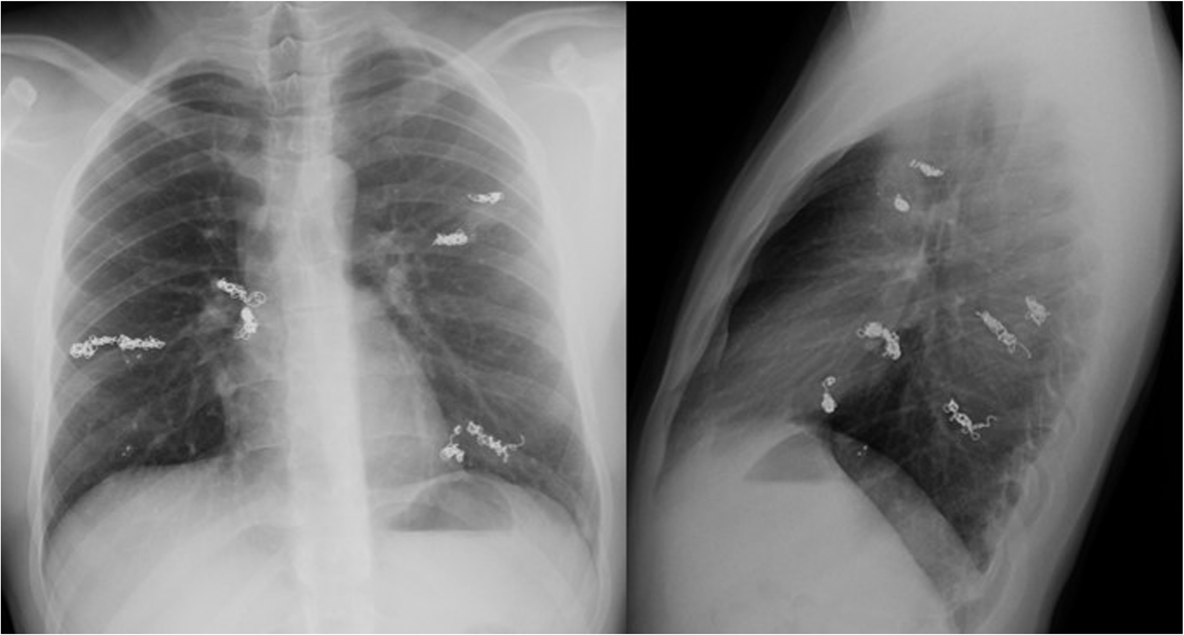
Chest X-radiograph showing previous material embolization but no signs of infection coming from the lower air pathway
